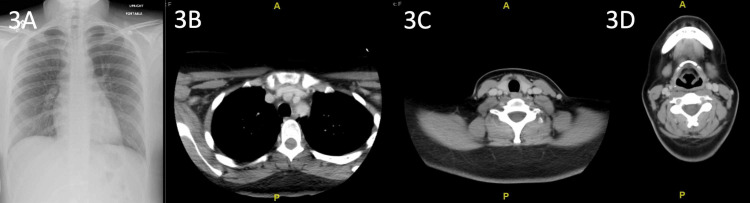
Repeat upright chest X-rays (AP view) and non-contrast CT of the chest and neck (soft tissue window) on the final hospital day The images show the resolution of pneumomediastinum in all previously noticed areas. There is no subcutaneous area present in the base of the left neck (3A), superior mediastinum (3B), along the carotid sheath (3C), or retropharyngeal space (3D) AP: anteroposterior; CT: computed tomography
